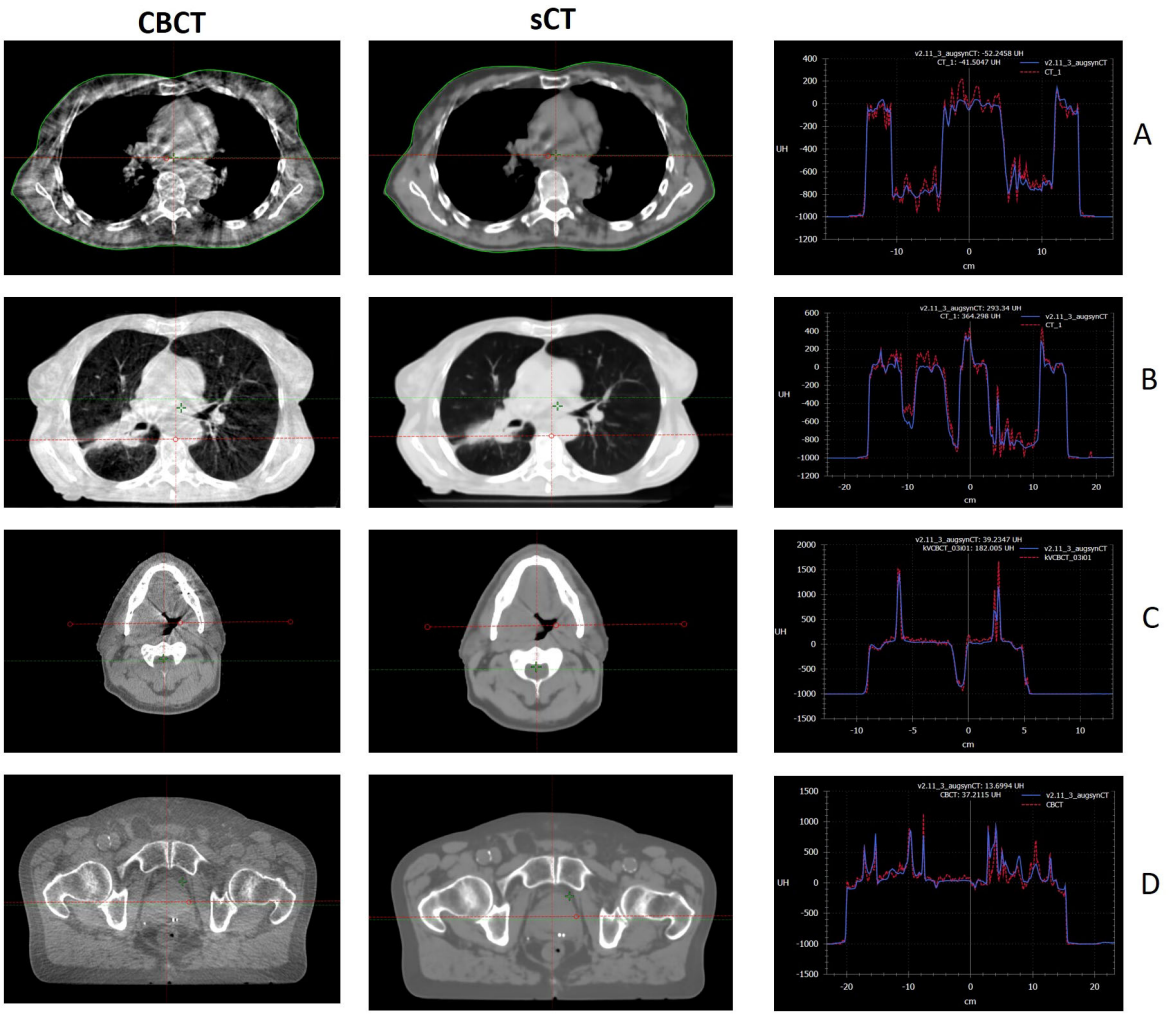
Example of sCT (right) and the CBCT (left) images for breast (A), thorax (B), head and neck (C) and male pelvis (D) anatomies. Example of Hounsfield unit comparison between sCT (blue line) and CBCT (red line).
